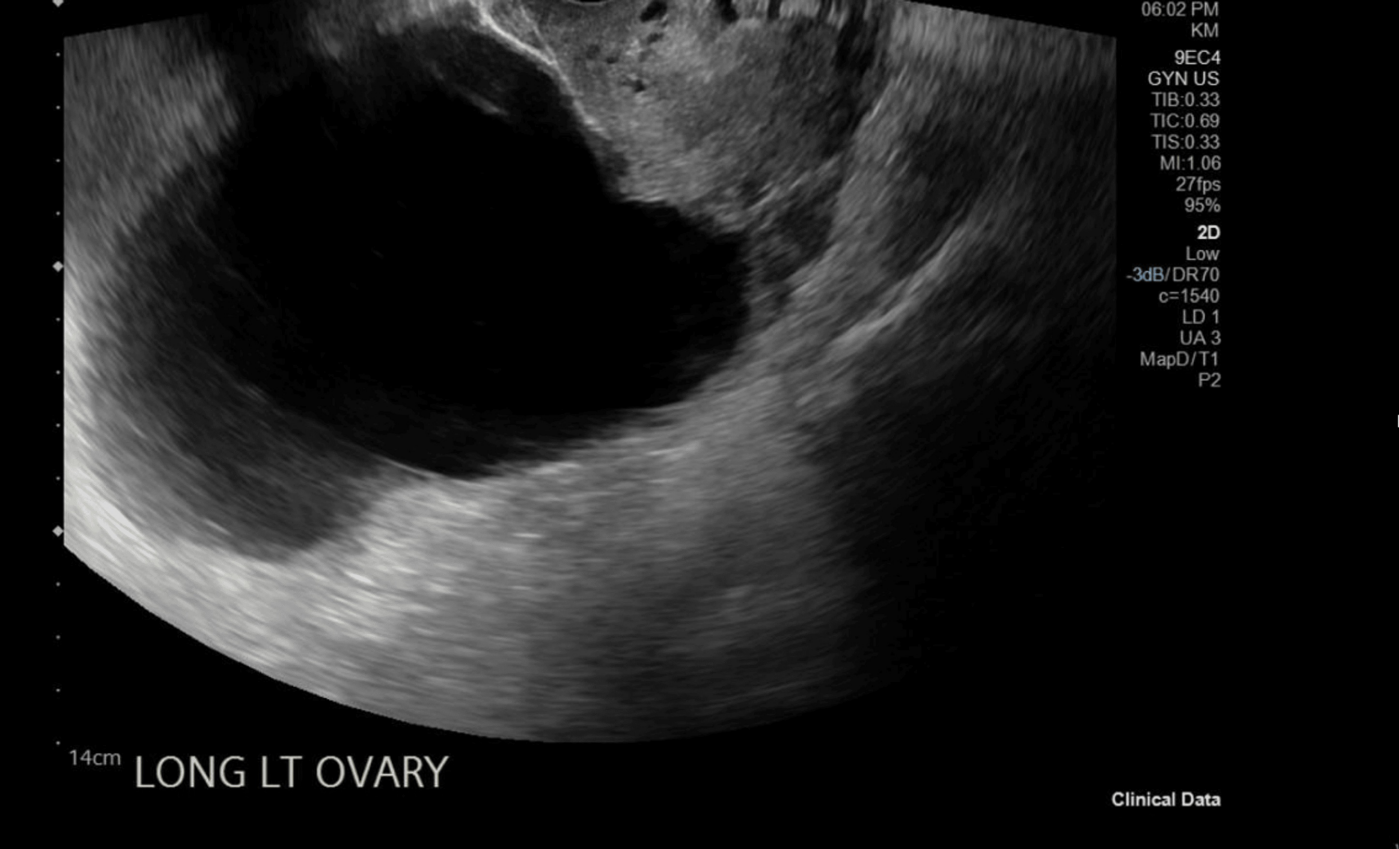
Transvaginal ultrasound imaging showing the left ovarian cyst
